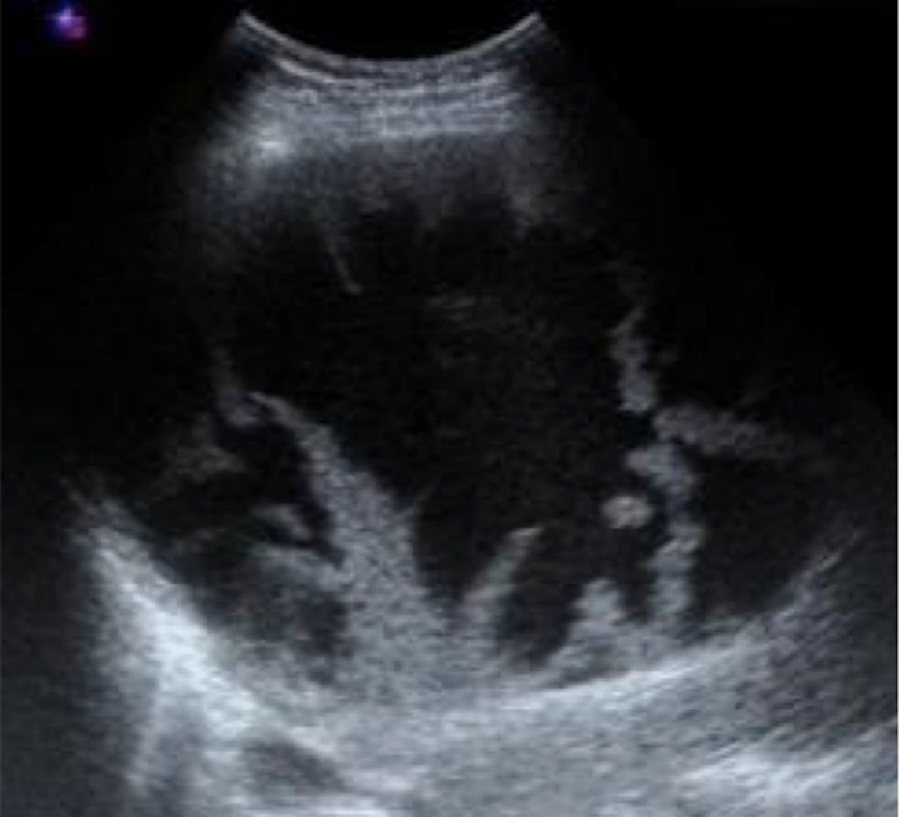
Subacute haemorrhage in a simple hepatic cyst in a 70-year-old male. Ultrasonography shows a spontaneous mobile area of hyperechogenicity inside the cyst, appearing as a “fern leaf”
